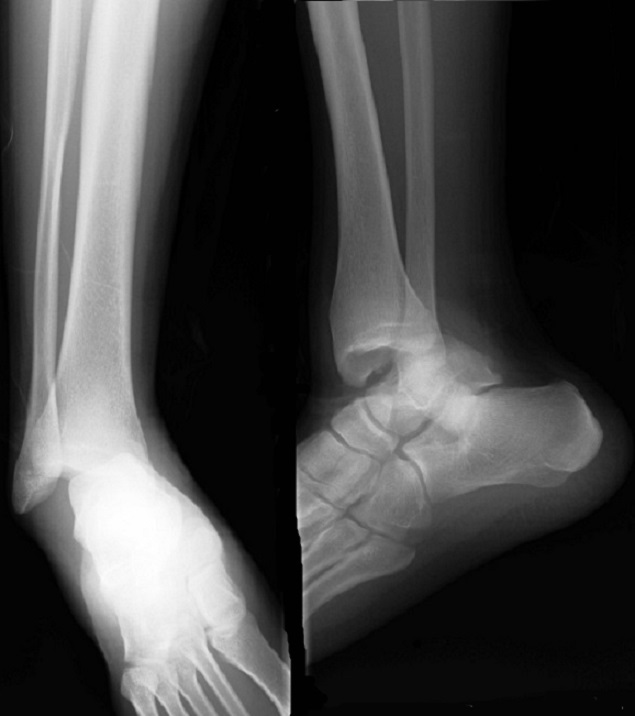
Radiographies initiales de la cheville face et profil montrant la luxation tibiotalienne pure
